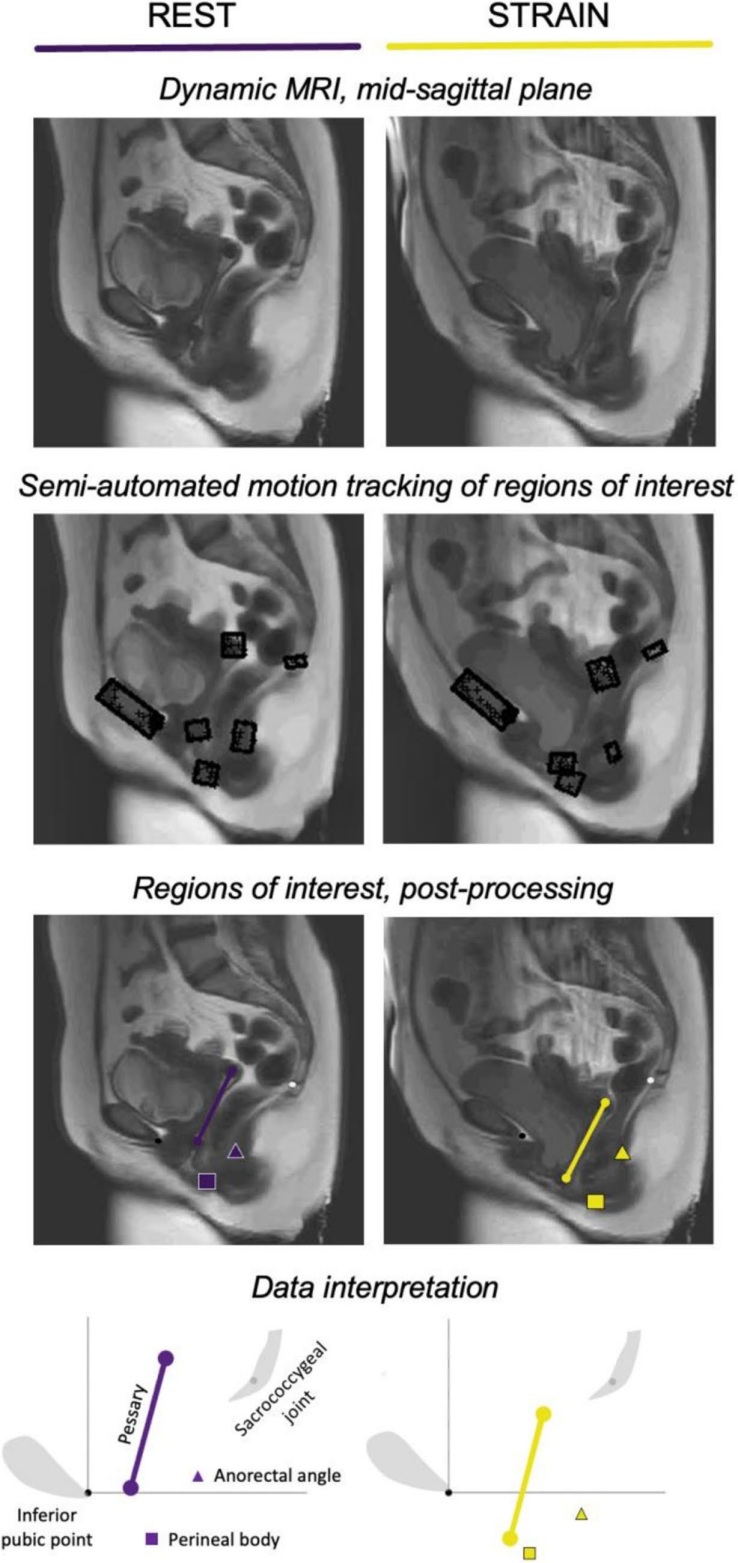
Application of customized motion-tracking software to track regions of interest on dynamic pelvic MRI and convert them into standardized coordinates for data analysis
